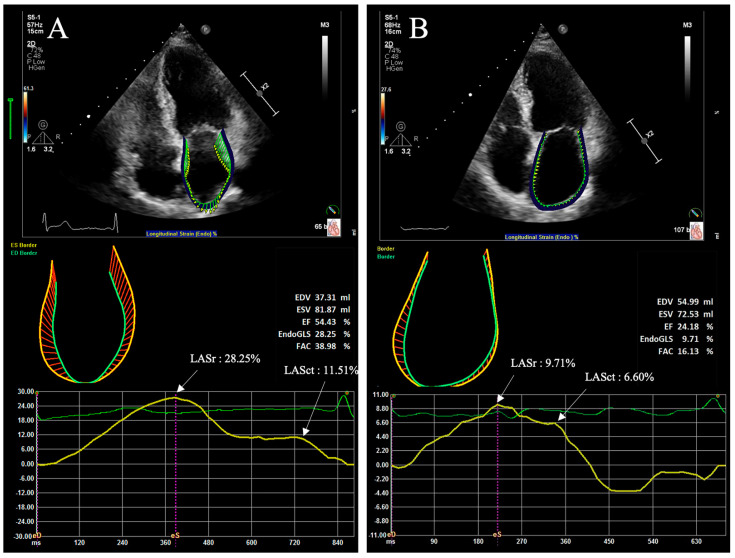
Representative measurements of LA strain by two-dimensional echocardiography. Standard apical four-chamber view images of 20 consecutive cardiac cycles were stored, and LA strain was measured in one of the cardiac cycles. (A) LASr and LASct in a subject with sinus rhythm; (B) LASr and LASct in a subject with AF.
